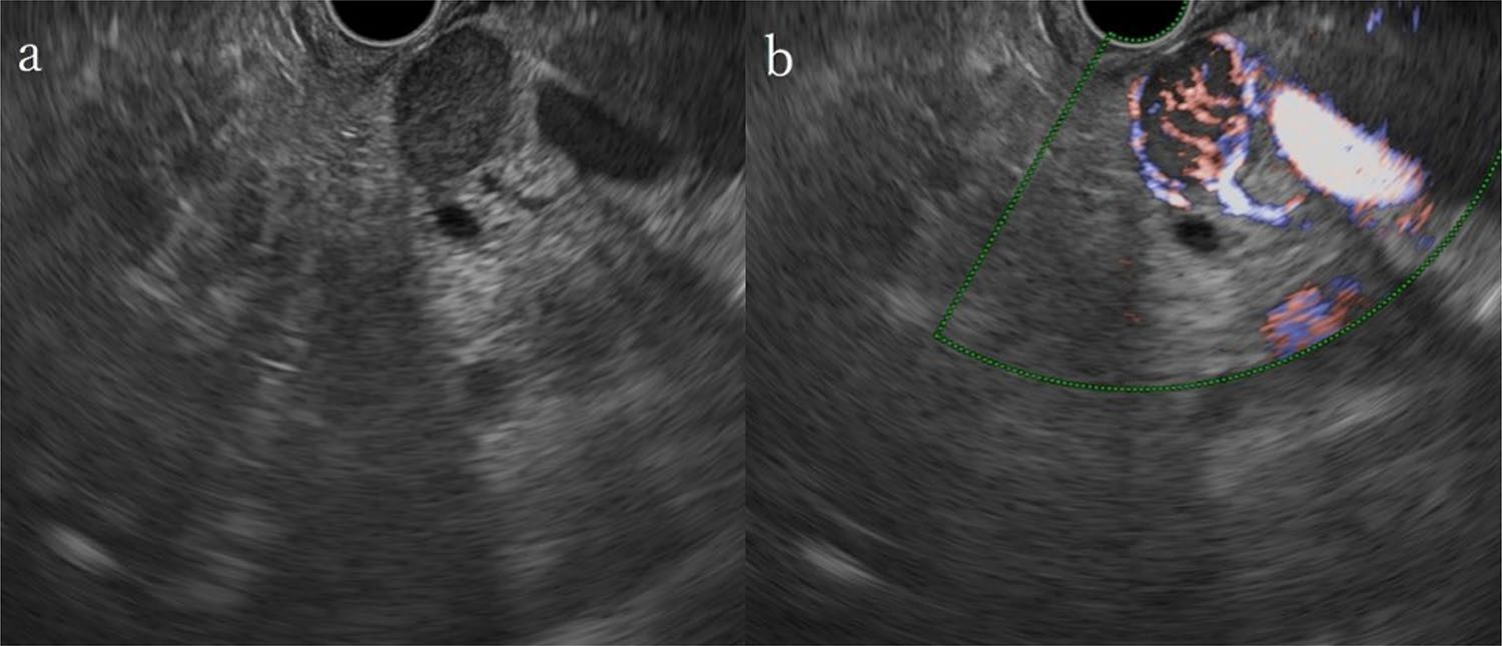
Endoscopic ultrasound showed a multiple well-defined hypoechoic masses in the pancreas, and b color Doppler ultrasound showed abundant blood flow inside the masses
